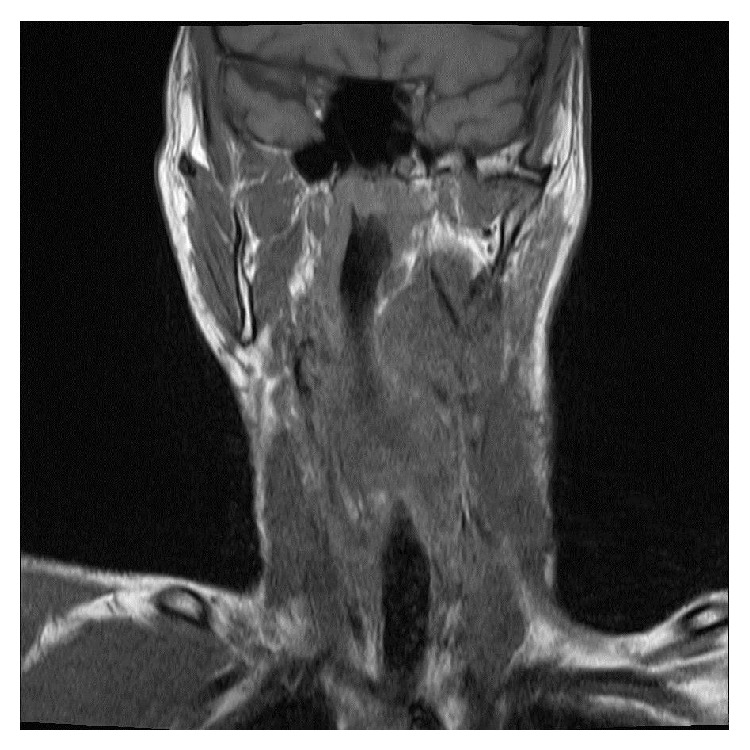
Coronal T1-weighted MRI showing the left pharyngeal mass with airway depression.
